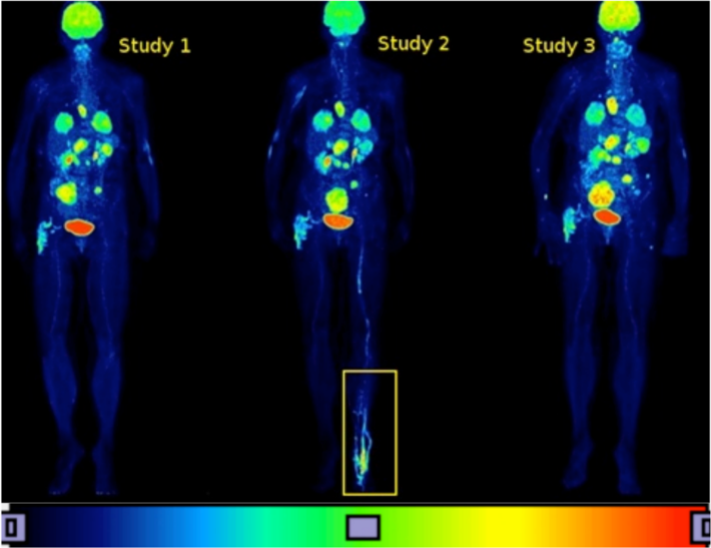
PET/CT images of patient P31 at the three stages. The patient presents high unusual uptake of the FDG biomarker in his/her left leg at the second stage of treatment, not related to the melanoma
